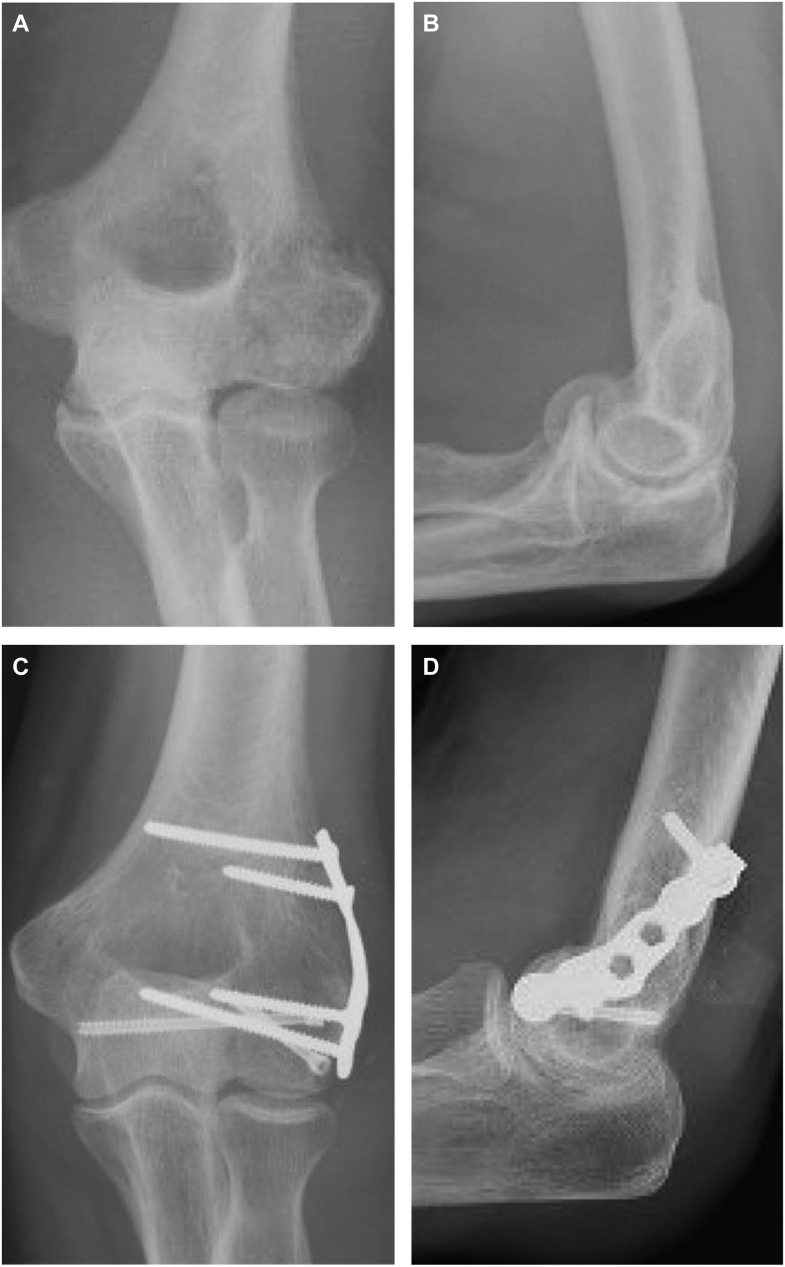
Radiographic images of a comminuted capitellum fracture (A and B) treated with headless compression screws and buttress plating (C and D).
